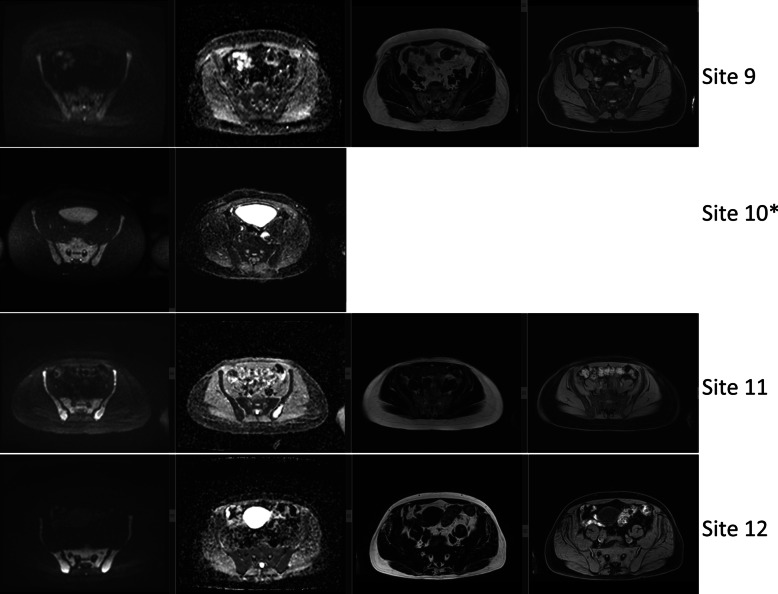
Initial MR images from each site. Axial images through the pelvis for DWI (b900, ADC) and T_1_-w Dixon (fat, water) sequences acquired on the first datasets (9 patients and 2 volunteers) from each site; lines correspond to scanner information listed in Table 1. No data are presented for site 8 as data were unavailable (incomplete). Volunteer data are marked by the * sign
